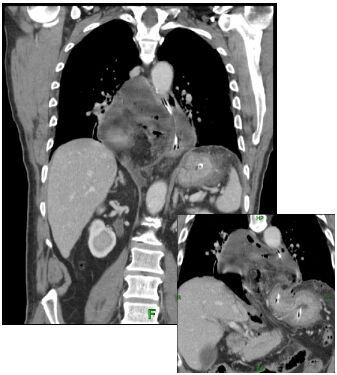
CT-scan. Coronal reconstruction CT-slice (3.7 mm). Intrathoracic mass consisting of mesenterial fat, free-intraperitoneal fluid and free-intraperitoneal air (1). On the left-side of this mass the esophagus is seen with a nasogastric tube (2) indicating a right-sided para-esophageal hernia with free intra peritoneal air and fluid. Intra-abdominal positioned stomach (3).
